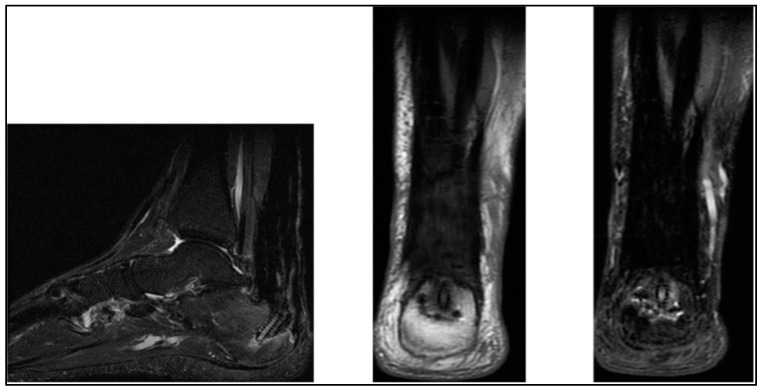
MRI view of Achilles tendon reconstruction.
